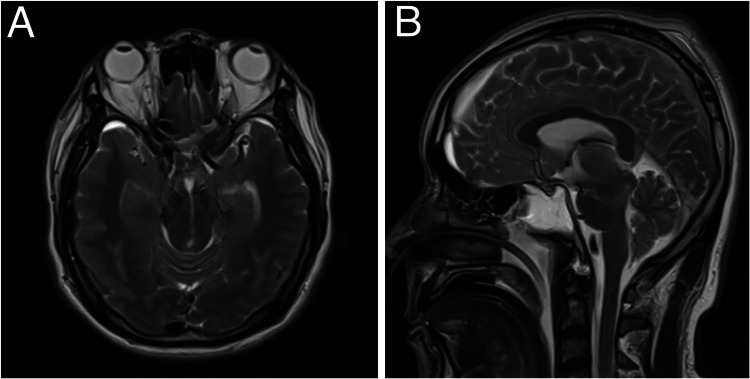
Non-contrast cranial MRI in (A) axial T2 and (B) sagittal T2 sequences showing compression of the bilateral midbrain and obliteration of the perimesencephalic sulcus.
